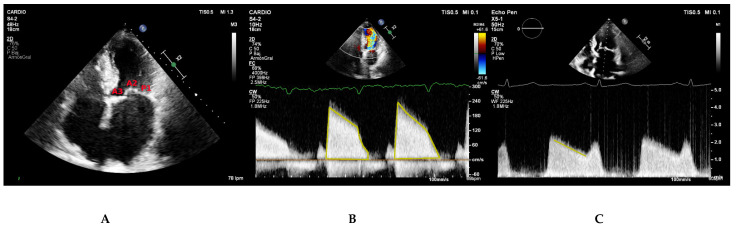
(A) Apical four chamber view depicting the P1, A2, and A3 mitral scallops. (B,C) Continuous wave Doppler parallel to the mitral inflow in apical four-chamber view. The Doppler profile (B) and the deceleration slope of the E wave (C) are traced to obtain the transmitral gradients and the PHT respectively.
